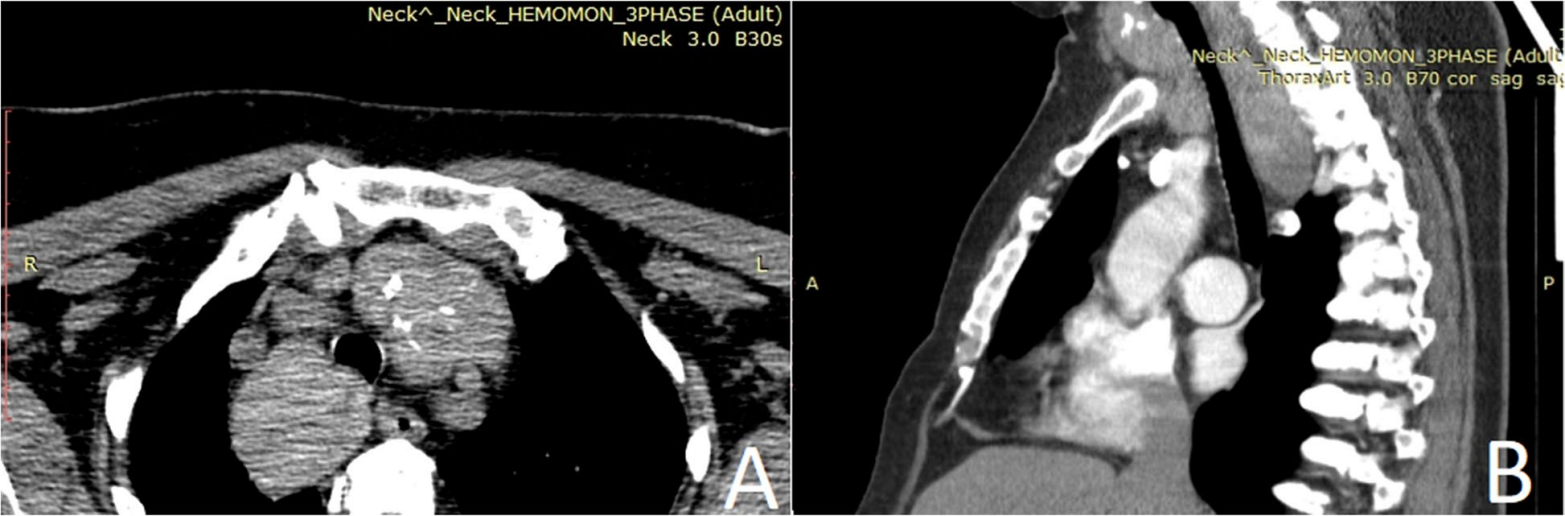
CT scan revealing a retrosternal goiter. A Axial image. B Sagittal image
